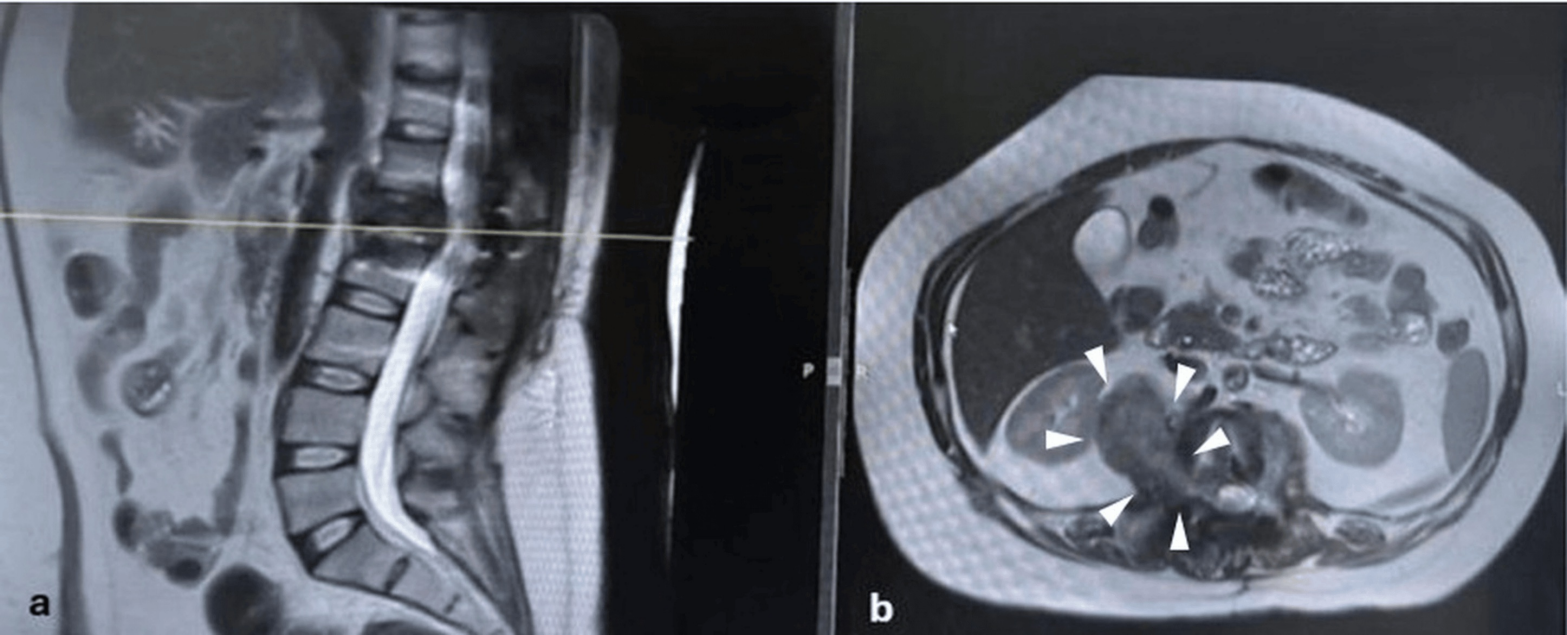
Sagittal and axial contrast-enhanced magnetic resonance imaging (MRI) of the lumbar spine at eight months follow-up a) Sagittal T2-weighted MRI demonstrating the lumbar spinal canal. The yellow reference line marks the level of the axial slice shown in panel b; b) Axial T2-weighted MRI at the corresponding level reveals a space-occupying lesion within the spinal canal (arrowheads), producing significant compression of the neural elements.
